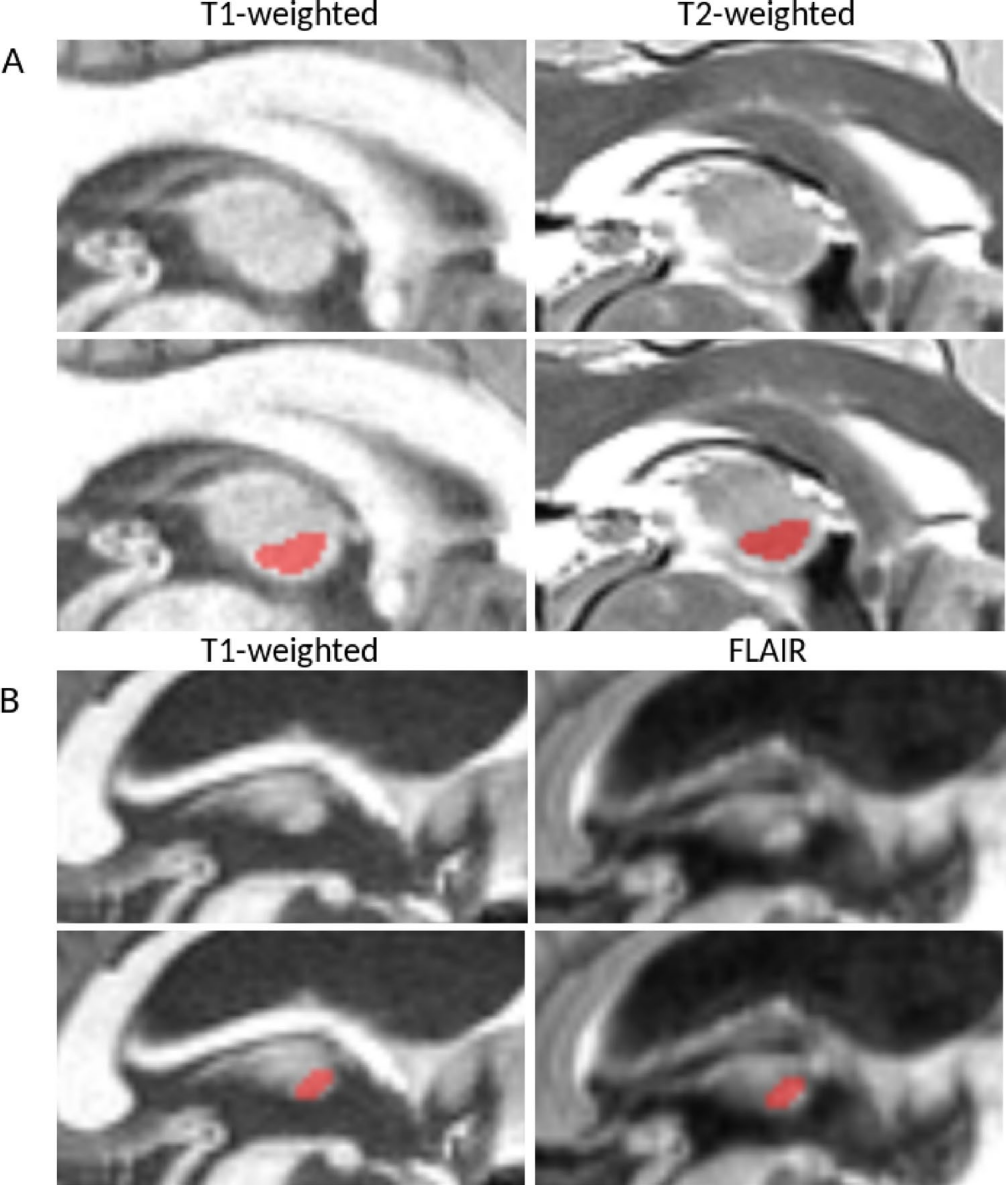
Contrast comparisons between sagittal slices of a T1w MRI and a coregistered sequence, including (A) T2w from the HCP Young Adult dataset (B) or FLAIR from the Toulouse dataset. The top row displays the original MRI slices without segmentation, while the bottom row presents the corresponding slices with manual IA segmentation to facilitate visualization
